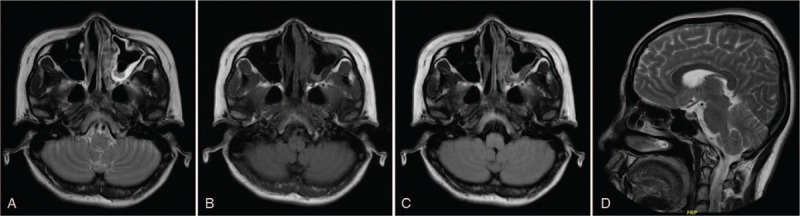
(A–D) Transverse and sagittal magnetic resonance imaging scan of the brain revealing abnormal signals in the left nasal cavity.
